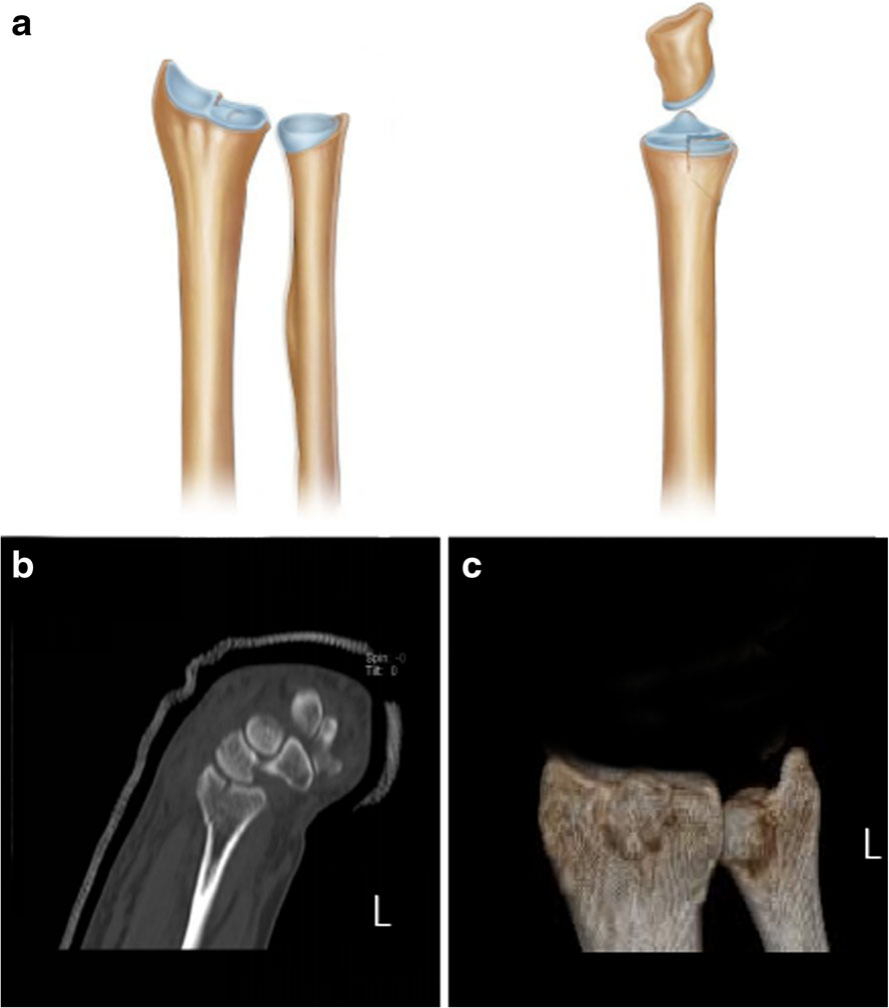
Dorsal depression fracture. a The dorsal half of the lunate fossa is depressed (*left*, anterior view; *right*, lateral view). b, c CT and three-dimensional CT (left) scans
